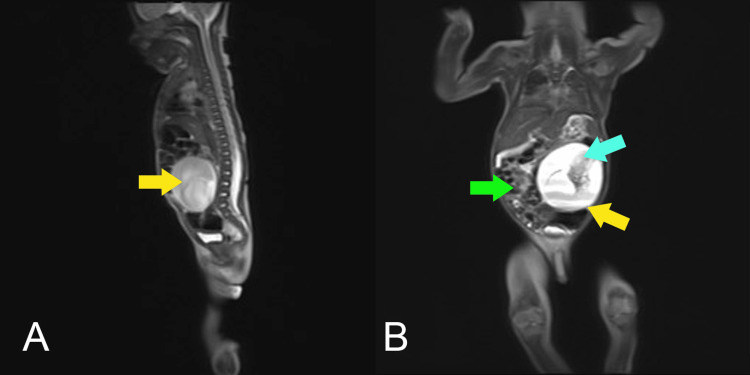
MRI of the patient showing a well-defined cystic mass in the left abdominal region anterior to the left kidney (A and B, yellow arrow) with a centrally located fetiform structure (B, blue arrow). The mass compresses and displaces the bowel loops anteriorly and to the right side (B, green arrow). MRI: magnetic resonance imaging
